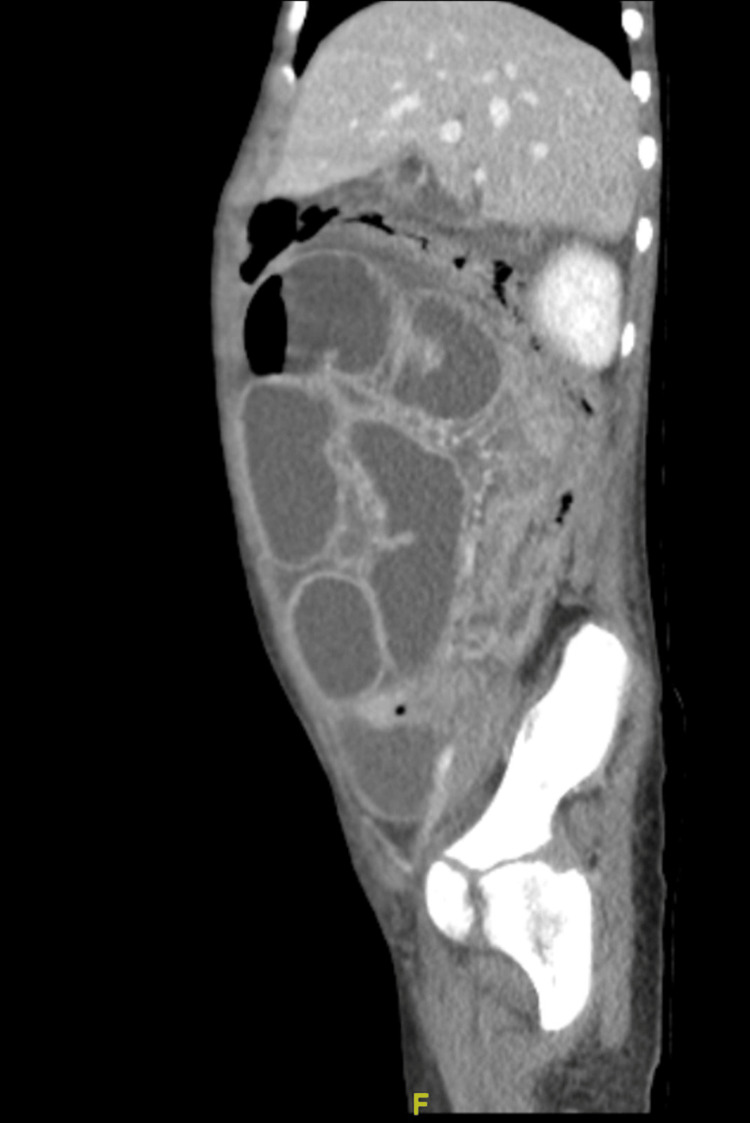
Sagittal plane of repeated CT scan of second Emergency Department presentation.
